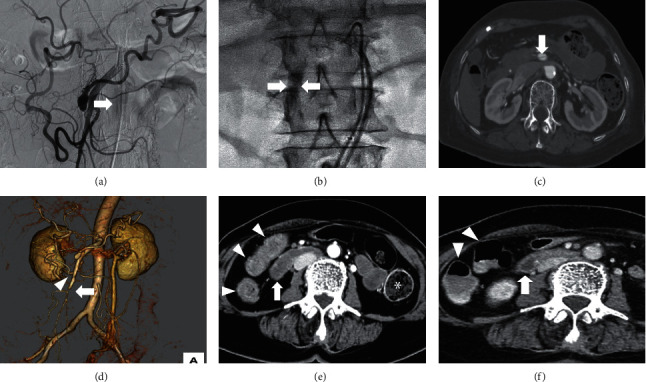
Radiology images of Case 2. A visceral artery aneurism is observed around the uncinate process of pancreas ((a), arrow). During selective cannulation to the aneurysmal neck, retention of the contrast medium is observed along the superior mesenteric artery ((b), arrows). CT shows separation of the inner and outer vascular layers ((c), arrow), confirming the diagnosis of superior mesenteric artery dissection. Stenosis of the roots of the ileocolic ((d), arrow) and right colic arteries ((d), arrowhead) is also observed. In contrast to the intact descending colon ((e), asterisk), mural thickening and delayed, reduced enhancement of the bowel wall are observed in the duodenum ((e), arrow) and ascending colon ((e), arrowhead). CT performed 14 days after angiography revealed improvement in mural thickening of the duodenum ((f), arrow), jejunum, and ascending colon ((f), arrowheads).
